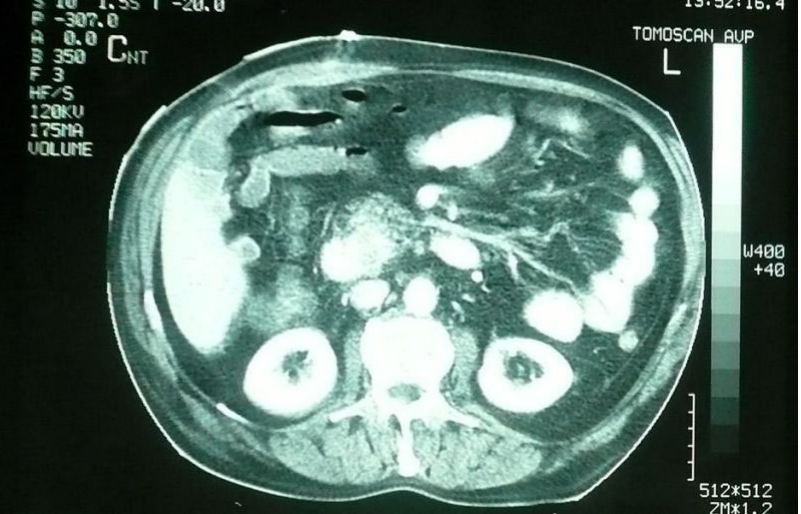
CT scan of the patient showing the tumor.
